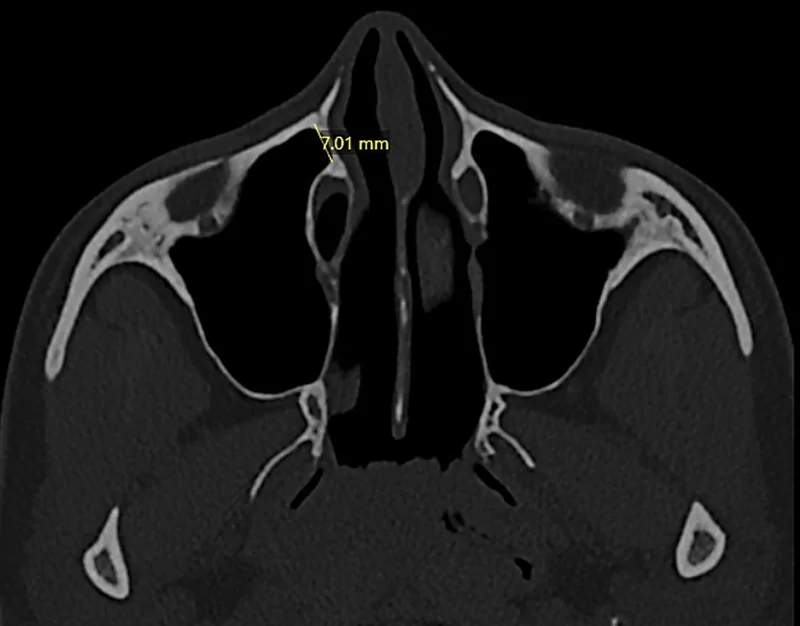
Computed tomography scan showing high grade feasibility of the TPRA in right healthy sinus type IIIb, with the yellow line representing the distance of the TPRA.
